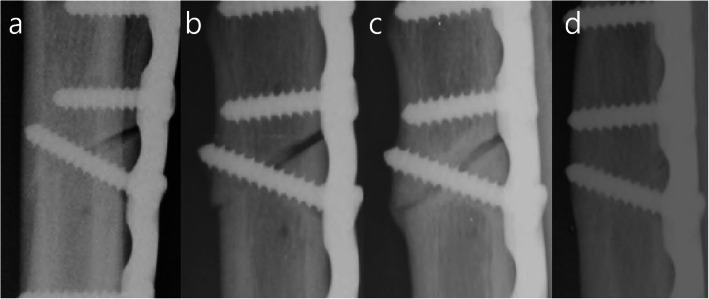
Serial radiographs of a freehand osteotomy with unparallel osteotomy surfaces and a long osteotomy reduction gap. a Immediate postoperative radiograph demonstrating that the lag screw could not compress a long osteotomy reduction gap. b After 2 months, bone defect still exists. c At 6 months, the osteotomy is united. d The complete osteotomy consolidation is achieved at 22 months
